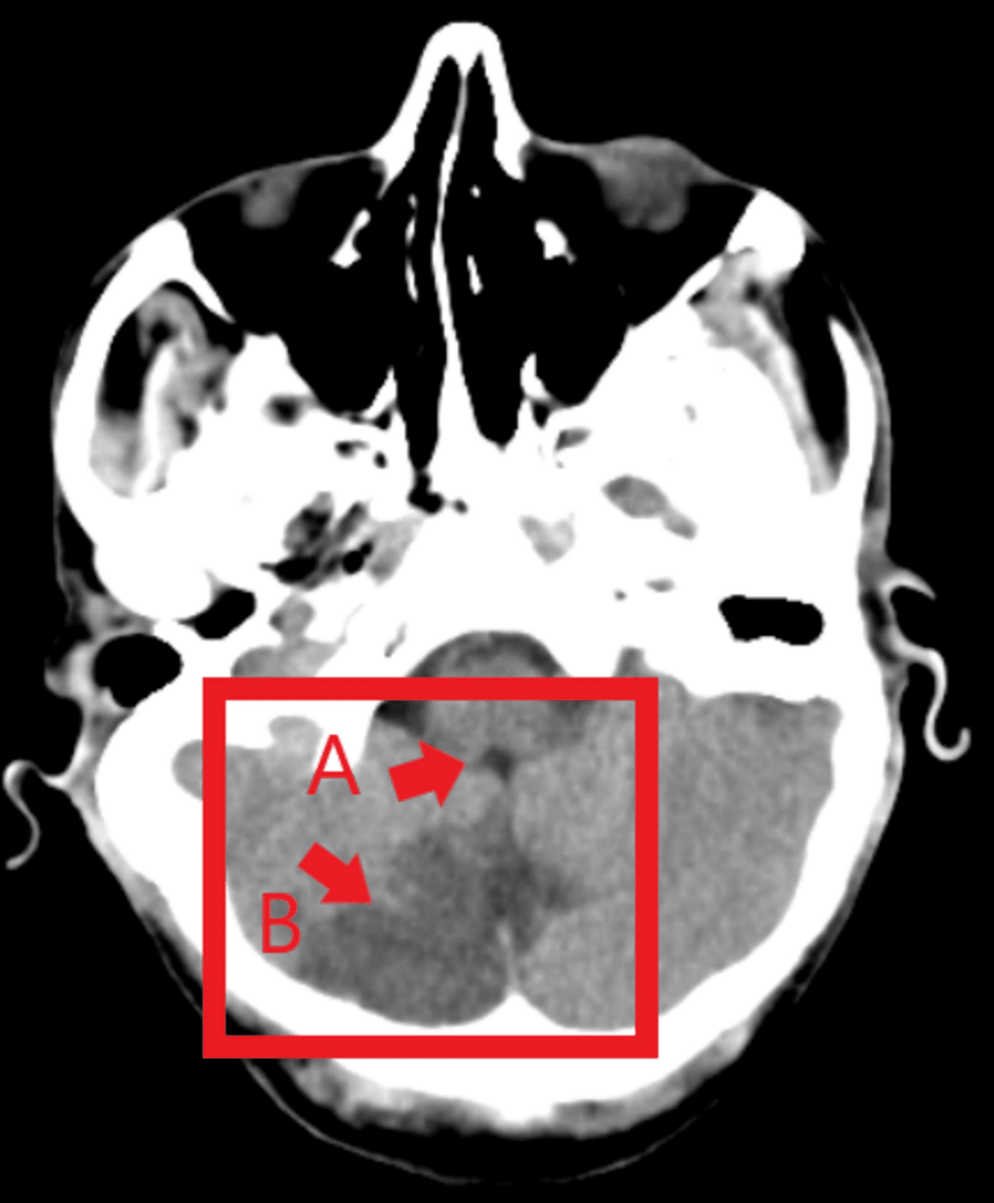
Computed tomography investigation of the patient A: Causing effacement of the fourth ventricle and cisterna magna. B: The presence of an ill-defined, hypodense area in the right cerebellar hemisphere.
